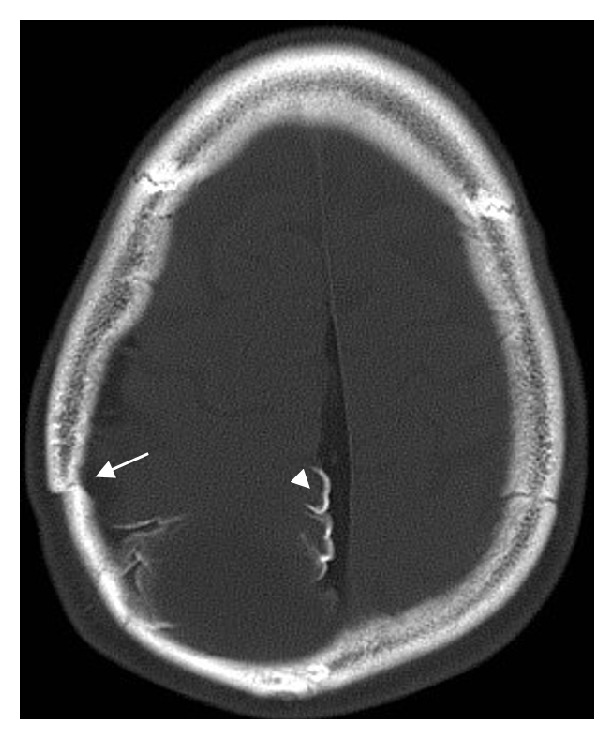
Noncontrast CT bone windows. Fracture of right parietal bone (arrow). Cortical gyriform calcifications are seen (arrowhead).
